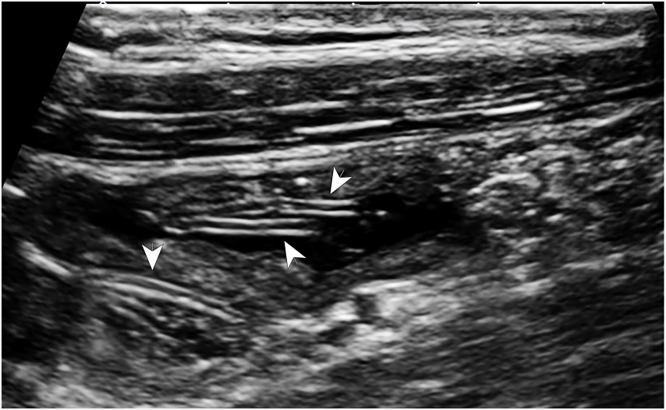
Abdominal ultrasonography revealing the characteristic double-lined hyperechoic linear structure of *Taenia* sp. within the small bowel (arrowheads).
